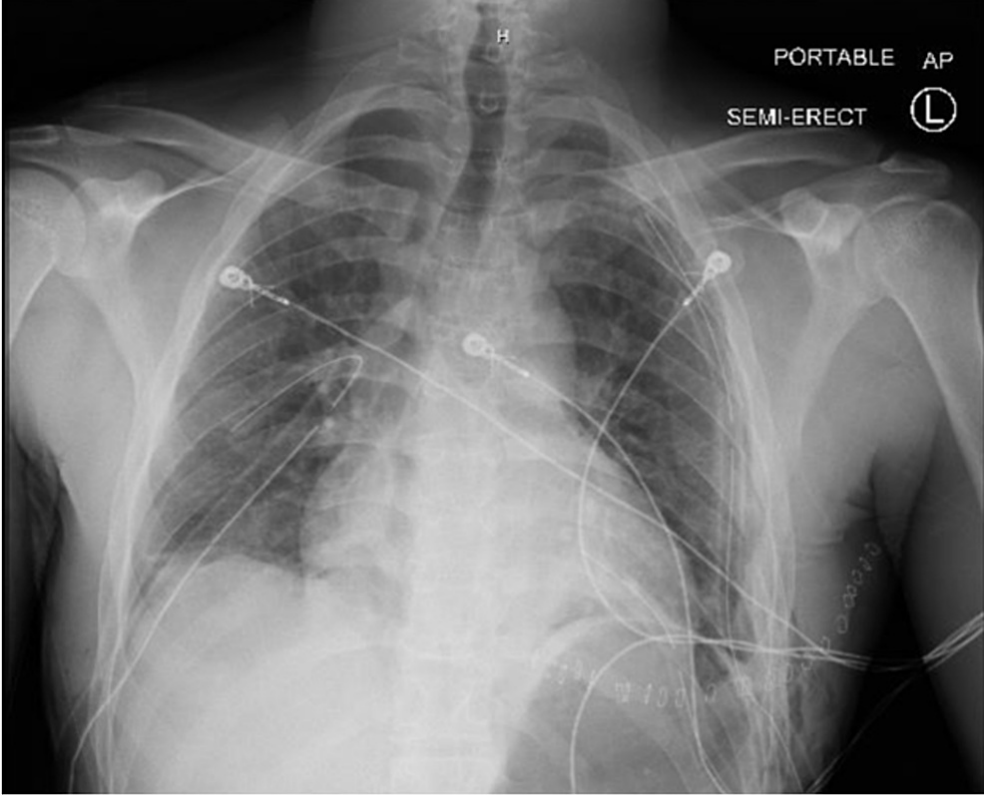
Postoperative chest x-ray demonstrating resolution of the pneumopericardium
